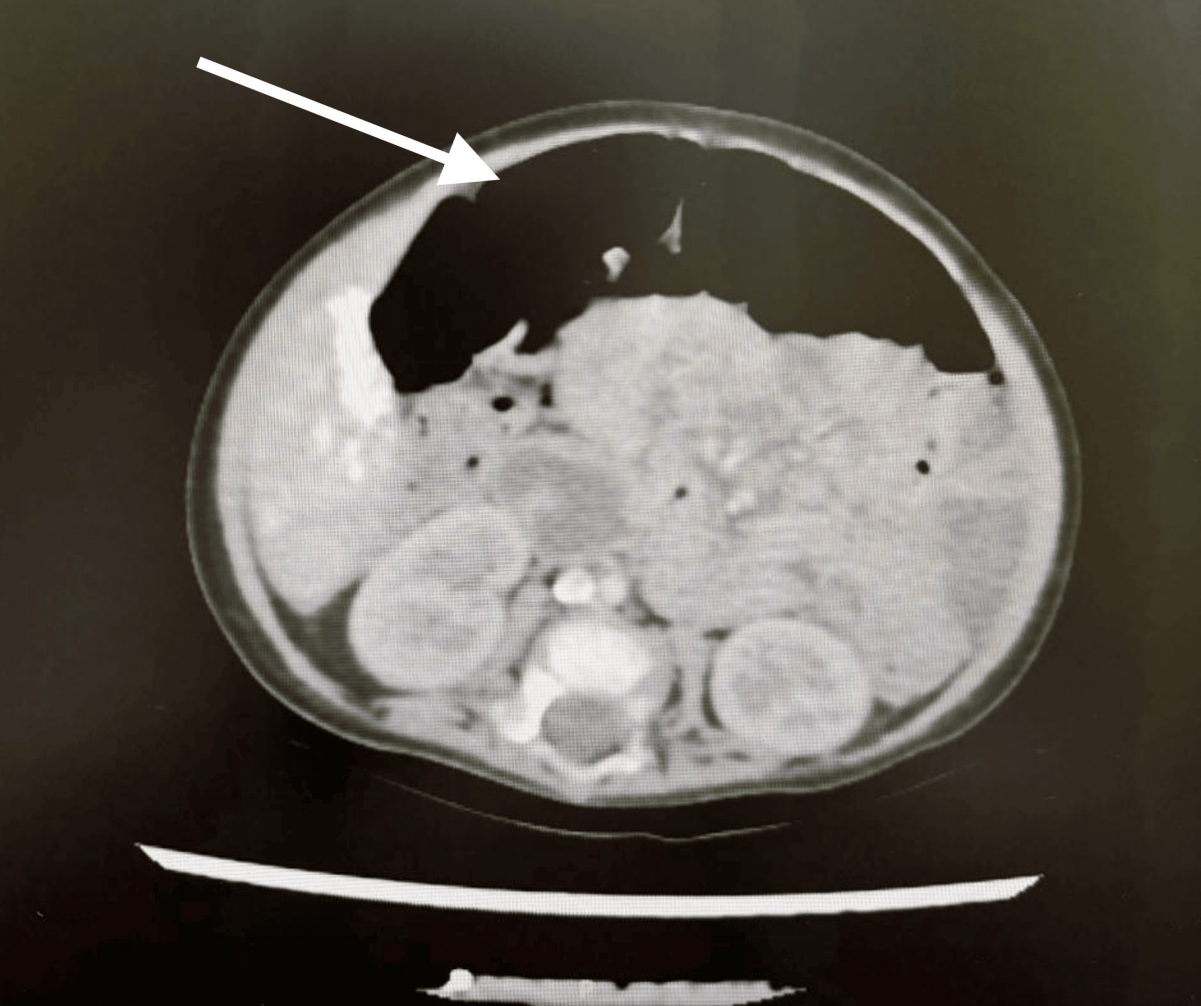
Abdominal CT scan obtained at seven months of age demonstrated complete pancreatic agenesis
